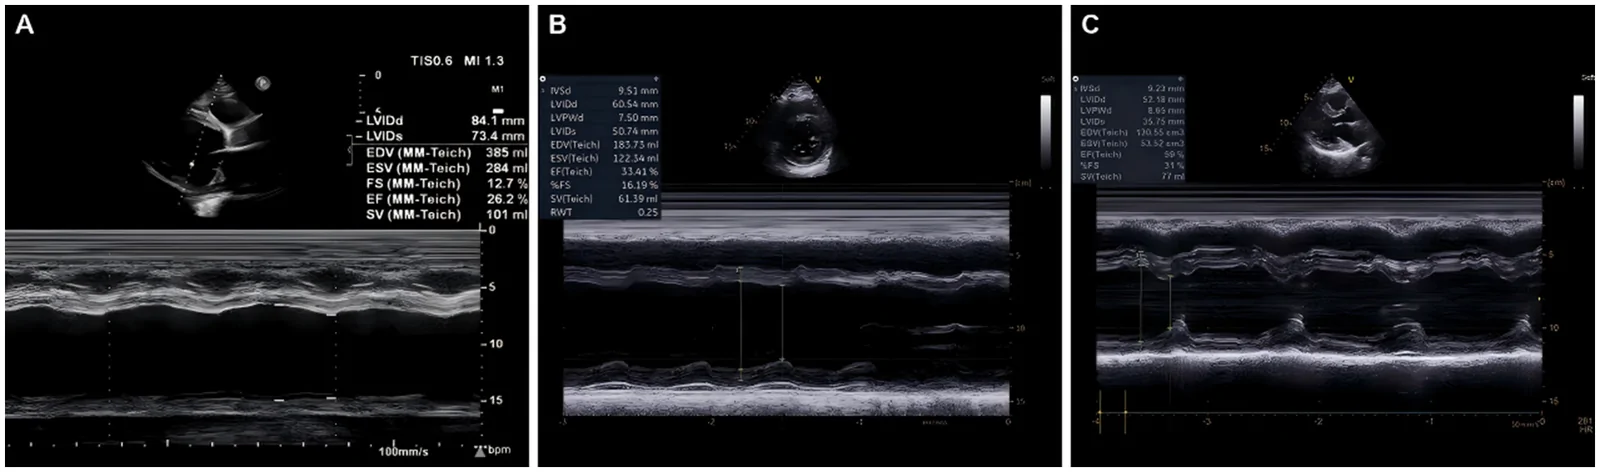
Serial transthoracic echocardiographic assessment of left ventricular systolic function. (A) At admission at 37 + 6 weeks of gestation, bedside echocardiography demonstrated severe left ventricular systolic dysfunction, with a LVEF of 26%. (B) On day 4 of VA-ECMO support, LVEF had increased to 33%, accompanied by hemodynamic stabilization and subsequent successful decannulation. (C) At the 5-year follow-up, LVEF was 59%, indicating sustained recovery of left ventricular systolic.
